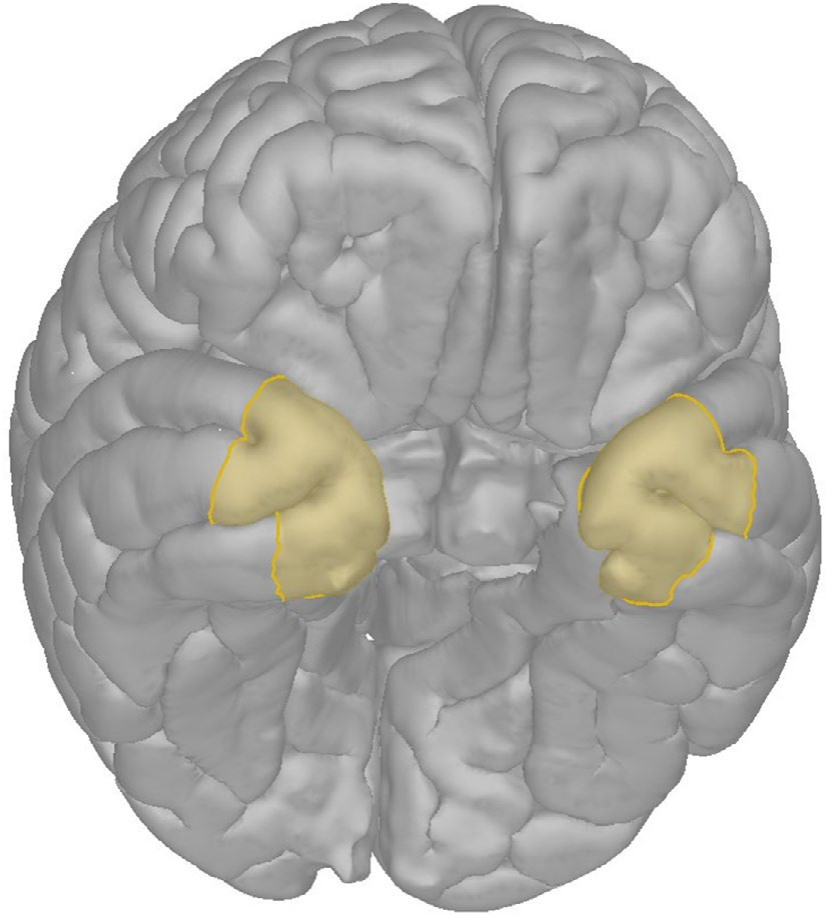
Temporal pole as segmented based on 36 MRI scans according to Fischl et al. ^12^ Note that there are no limiting lateral or basal sulci delineating the pole but rather the anterior end of the superior lateral sulcus as a landmark for segmentation.
